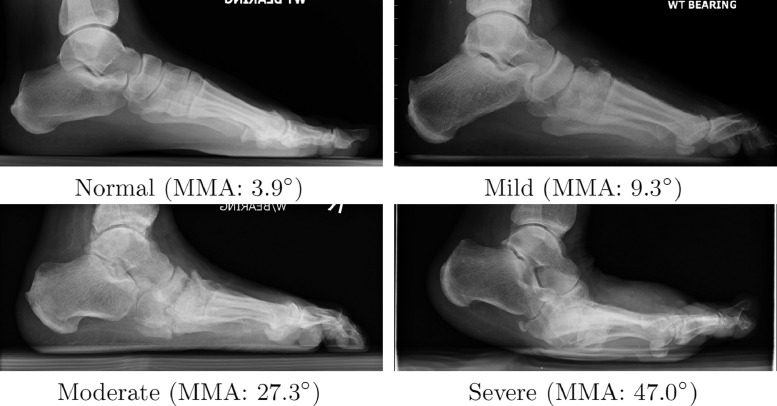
Examples of varying degrees of foot collapse based on Meary's angle (MMA = mean Meary's angle over all five clinical experts). The same images are classified as *normal, mild, severe* and *severe* based on clinical review. All radiographs have been cropped for better visualisation.
